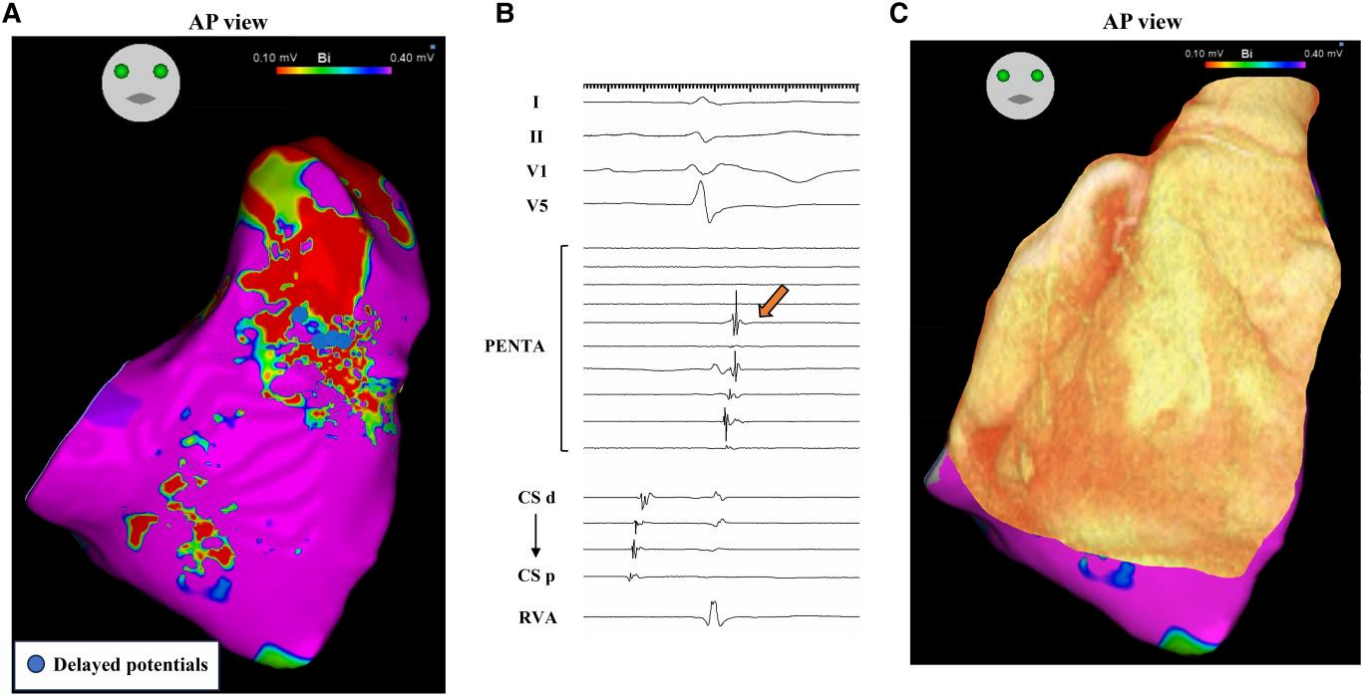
(*A*) Voltage mapping during SR. Delayed potentials are located within the dense scar area in the right ventricular free wall (tag). (*B*) Cardiac tracing of delayed potentials during the sinus rhythm is shown (arrow). (*C*) Three-dimensional computed tomography merged with the voltage mapping during SR. AP, anterior-posterior; DPs, delayed potentials; SR, sinus rhythm.
